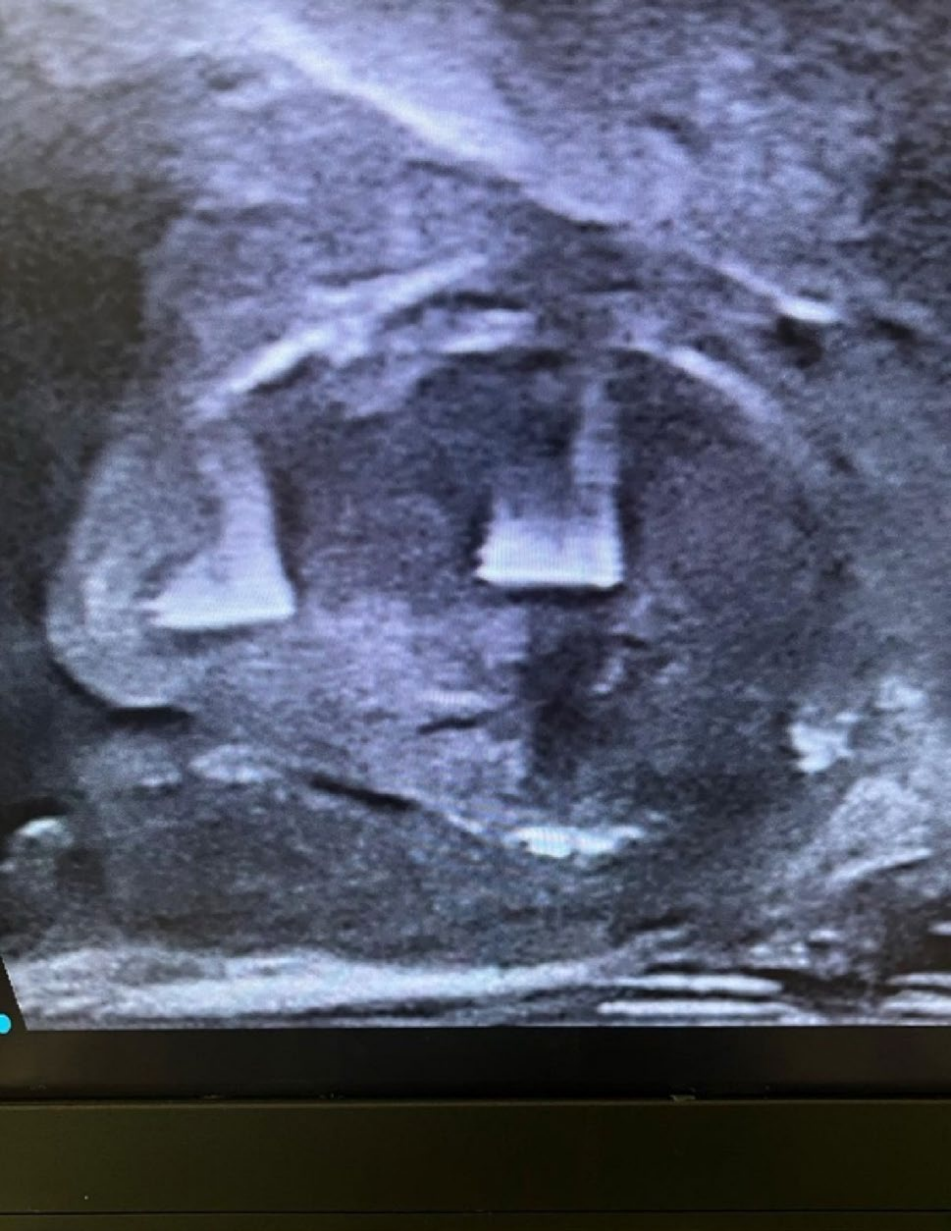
Transrectal ultrasound showing the prostatic Urolift clips prior to Aquablation
